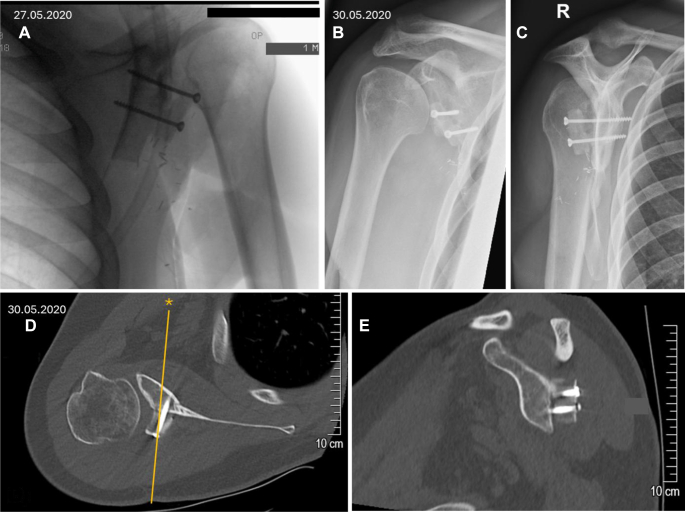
(A) Intraoperative fluoroscopy of the vascularized scapular bone flap and screw fixation. (B and C) Post-operative x-ray of the right shoulder after vascularized scapular bone flap and screw fixation. (D and E) Post-operative axial CT image demonstrating successful placement of the scapular bone flap. *CT*, computer tomography.
